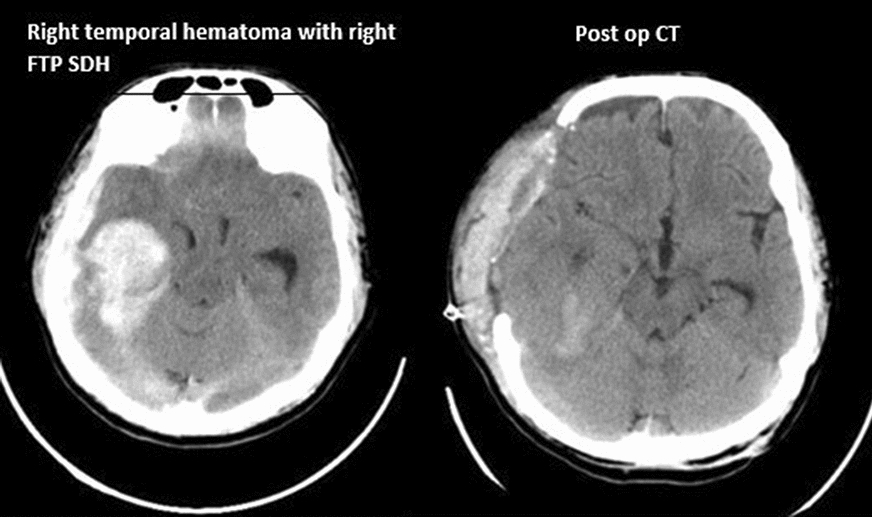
Right temporal hematoma with right FPP SDH and post op CT
